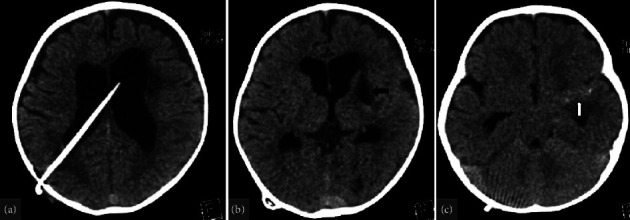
Axial brain CT scan without contrast taken during the second admission: (a) mild hydrocephalus with a ventriculoperitoneal (VP) shunt in the left lateral ventricle, (b) left lentiform nucleus encephalomalacia, and (c) arterial calcification along the left M1 segment of the middle cerebral artery (MCA).
